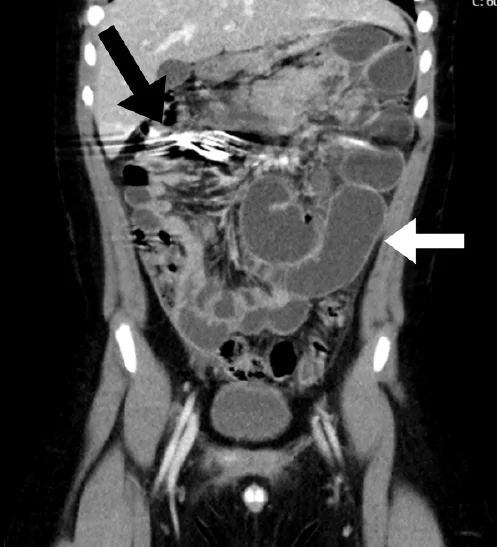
Computed tomography demonstrating streak artifact from ingested foreign bodies (black arrow) and dilated loops of bowel (white arrow) suggesting small bowel obstruction in a 4-year-old boy presenting with abdominal pain.
